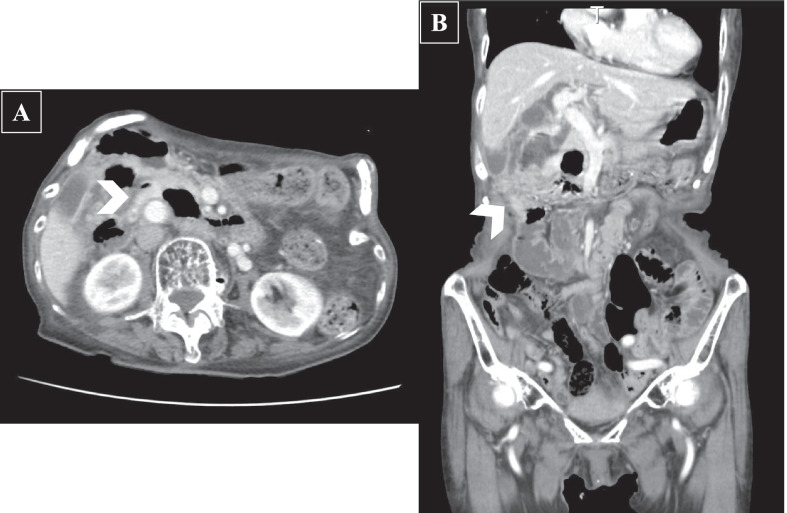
Contrast material-enhanced computed tomography. A Axial contrast-enhanced CT shows enhancement of thickened duodenal wall (*arrowhead*). B Reformatted coronal contrast-enhanced CT image also detects the thickened duodenal wall with inflammation involving periduodenal fat (*arrowhead*)
